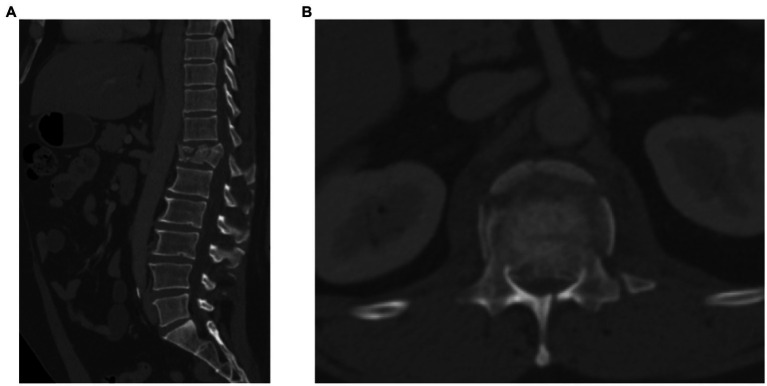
CT scan images of thoracolumbar spine demonstrating spinal fracture. (A) Sagittal CT scan showing T12 fracture with comminuted vertebral body. (B) Axial CT scan at T12 level demonstrating violation of the posterior cortex of vertebral body with retropulsion of bone fragments.
